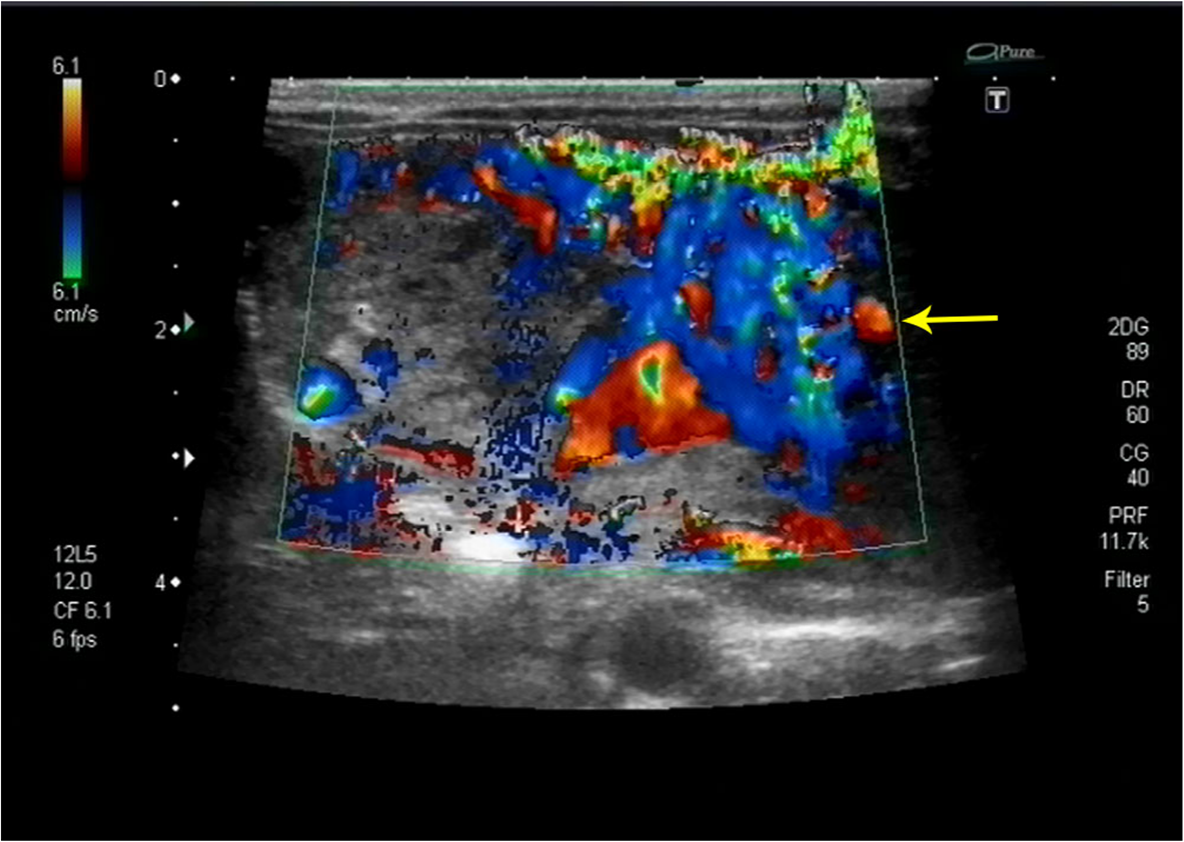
Views of the IHH from contrast-enhanced CT (Contrast-enhanced computed tomography). The left hepatic lobe is irregularly enhanced with a slightly lower density and the lesion shows peripheral enhancement of centripetal fill-in on arterial phase
